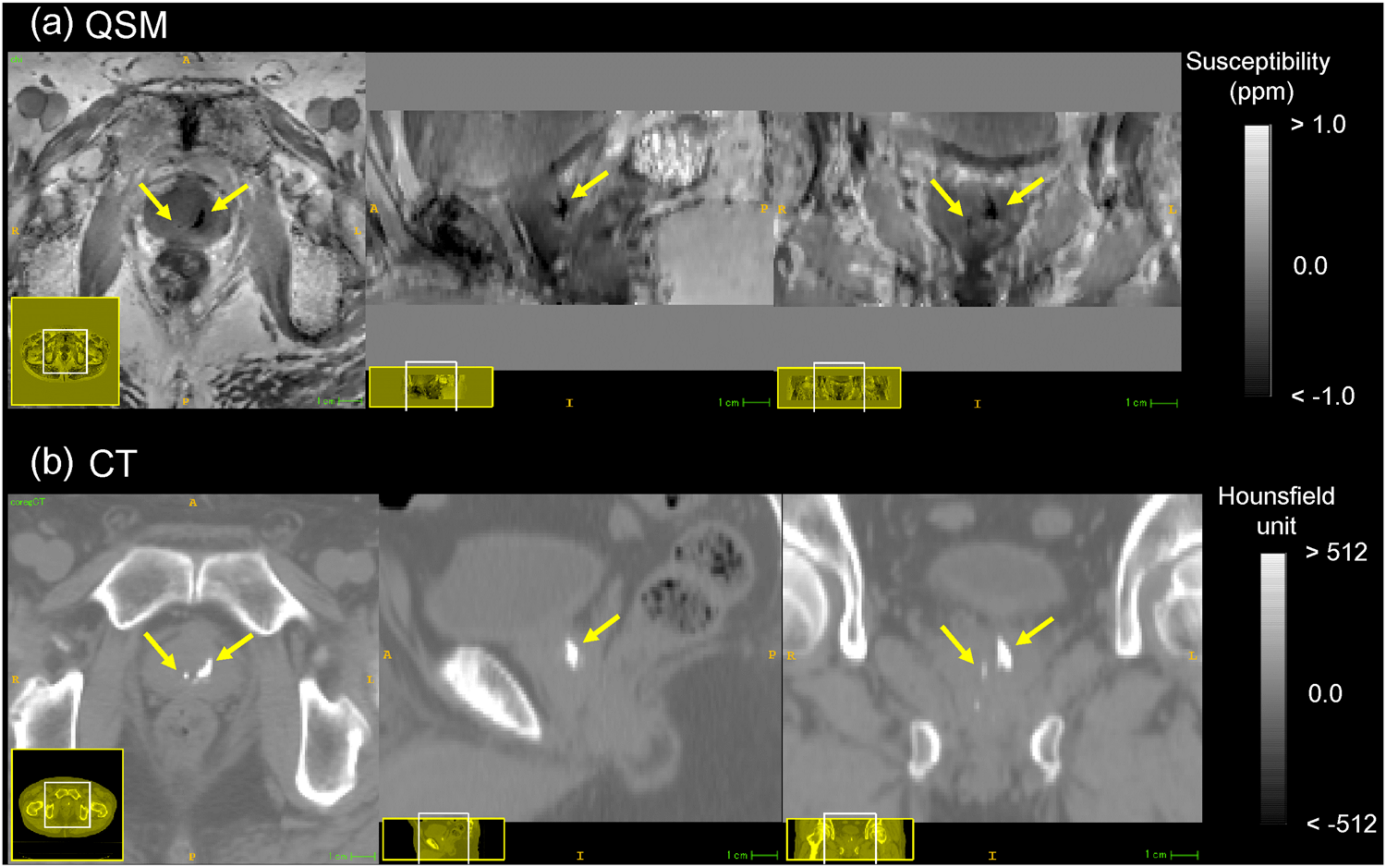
Representative prostatic calcifications (yellow arrow) in (a) quantitative susceptibility mapping and (b) computed tomography
